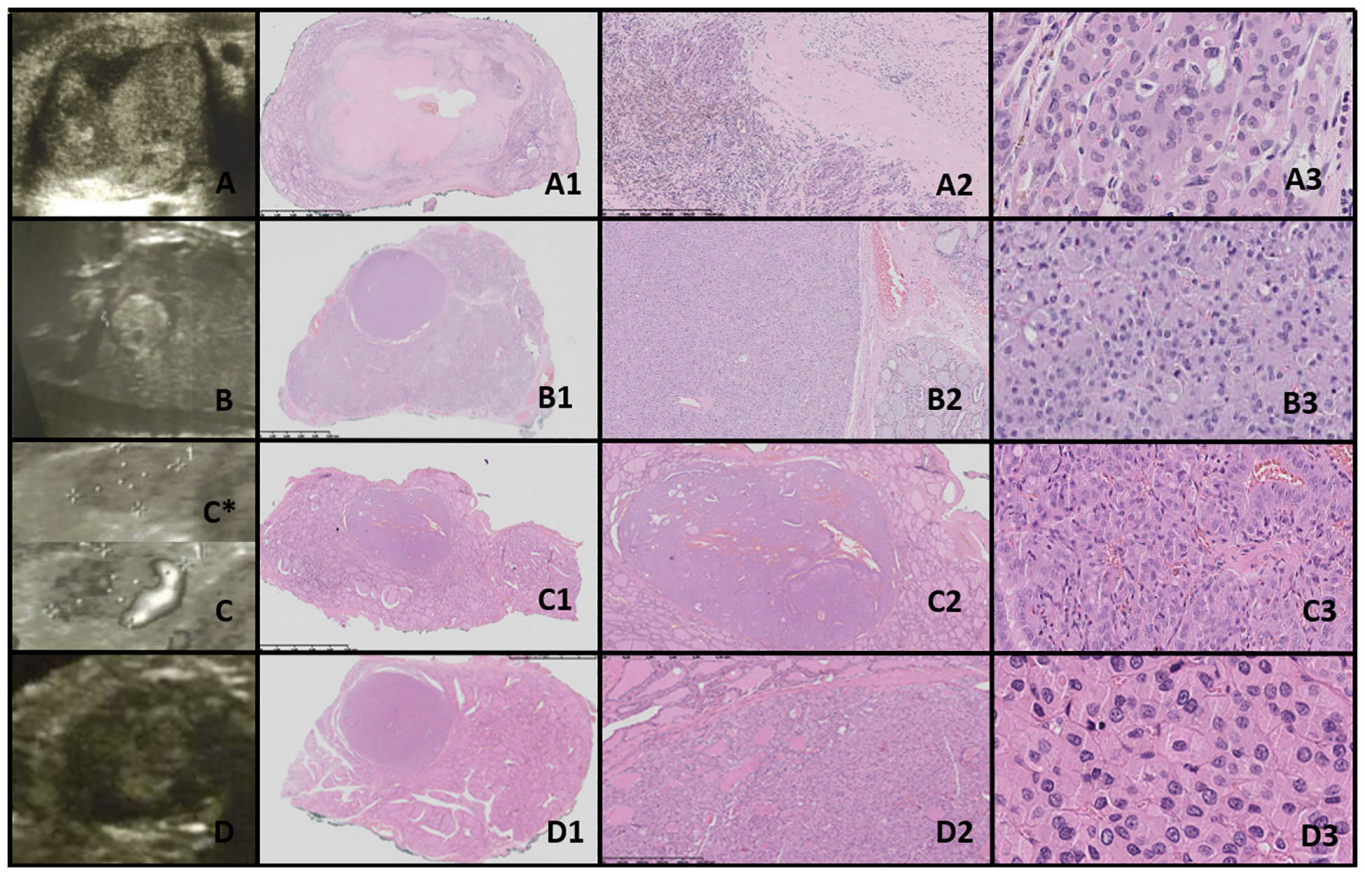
Oncocytic cell adenoma (OCA; Hürthle cell adenoma). Columns represent US and HE (magnification: A1–D1 ×5, A2–D2 ×50, and A3–D3 ×5,000). (A) Seventeen-year-old male patient with goiter; (B) 16-year-old female patient with a nodule found on US; (C) 17-year-old male patient with a nodule found on US; (D) 16-year-old male patient with a nodule found on US. US (C, D with power doppler, C*-without power doppler) reveals hyperechogenic nodules with small foci of hypoechogenic areas and increased mixed-type vascularity. HE examination reveals densely packed eosinophilic cells, and the hypoechogenic foci represent granular inflammatory tissue. Some of the cases might present with advanced fibrosis or contain medium-sized vessels (HE) and hyperperfusion in US (A, C, particularly). Cells are pleomorphic, with enlarged nuclei with prominent nucleoli.
